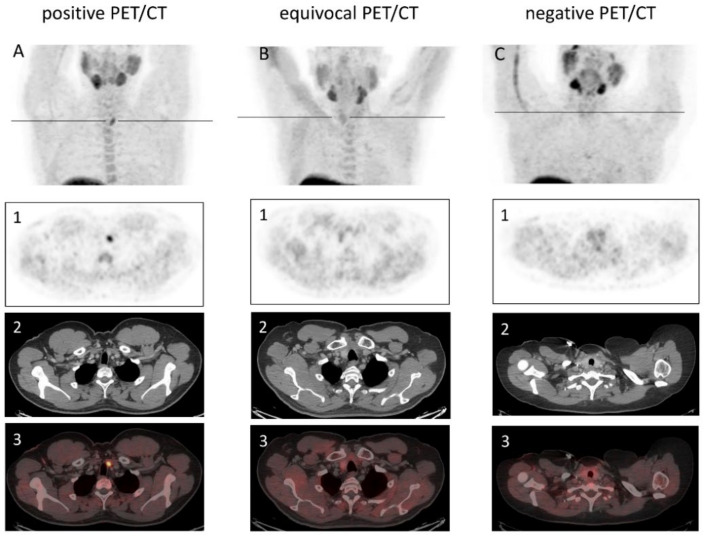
Patient examples of positive (A), equivocal (B), and negative (C) ^18^F-Fluorocholine PET/CT; PET (1), CT (2), and fused images of PET and CT (3). (A) a focal choline uptake correlating to a contrast enhanced lesion in the caudal left thyroid bed was detectable. (B) only weak and diffused choline uptake of the right thyroid tissue was visual. (C) no enhanced choline uptake was detectable.
